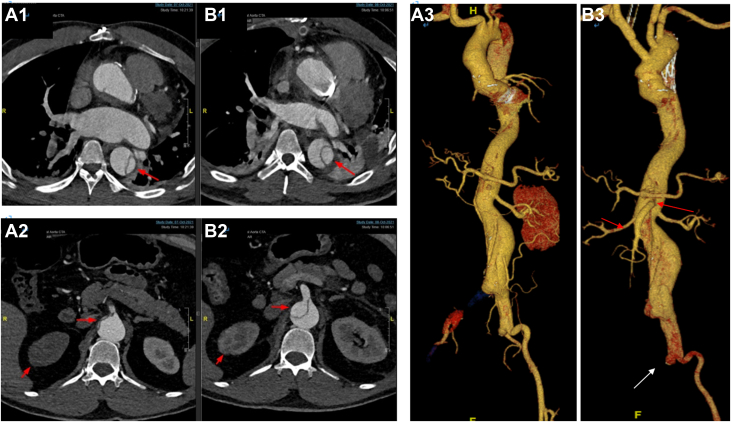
Dissection-Related Branch Vessels Before and After Open Repair (OR) Surgery (A1-A3) Computed tomography angiography results before OR. (B1-B3) Results following OR. Red arrows (A1 and B1): the true lumen is more widely opened after than before OR. Red arrows (A2 and B2): the true lumen of the superior mesenteric artery is more open after OR, with blood flow improvement, than before OR. Contrast imaging of the right kidney regained after OR. Red arrow (A3 and B3): Three-dimensional reconstruction indicate superior mesenteric and right kidney artery were visualized following OR; white arrow (B3): The right iliac artery continues to show no blood flow.
